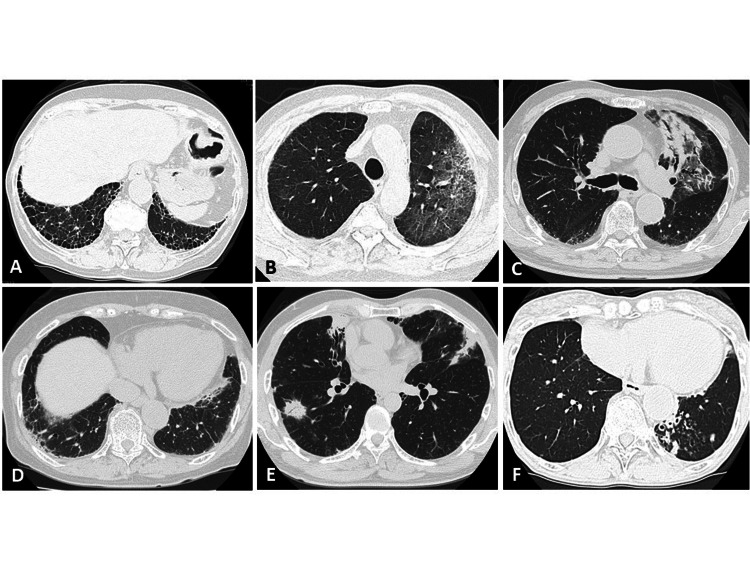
Representative radiological finding on thoracic CT Representative thoracic computed tomography findings for MPA, (A) showing honeycombing, (B) ground-glass opacities, (C) consolidation, (D) reticular shadowing, (E) nodules, and (F) bronchiectasis with centrilobular nodules.
